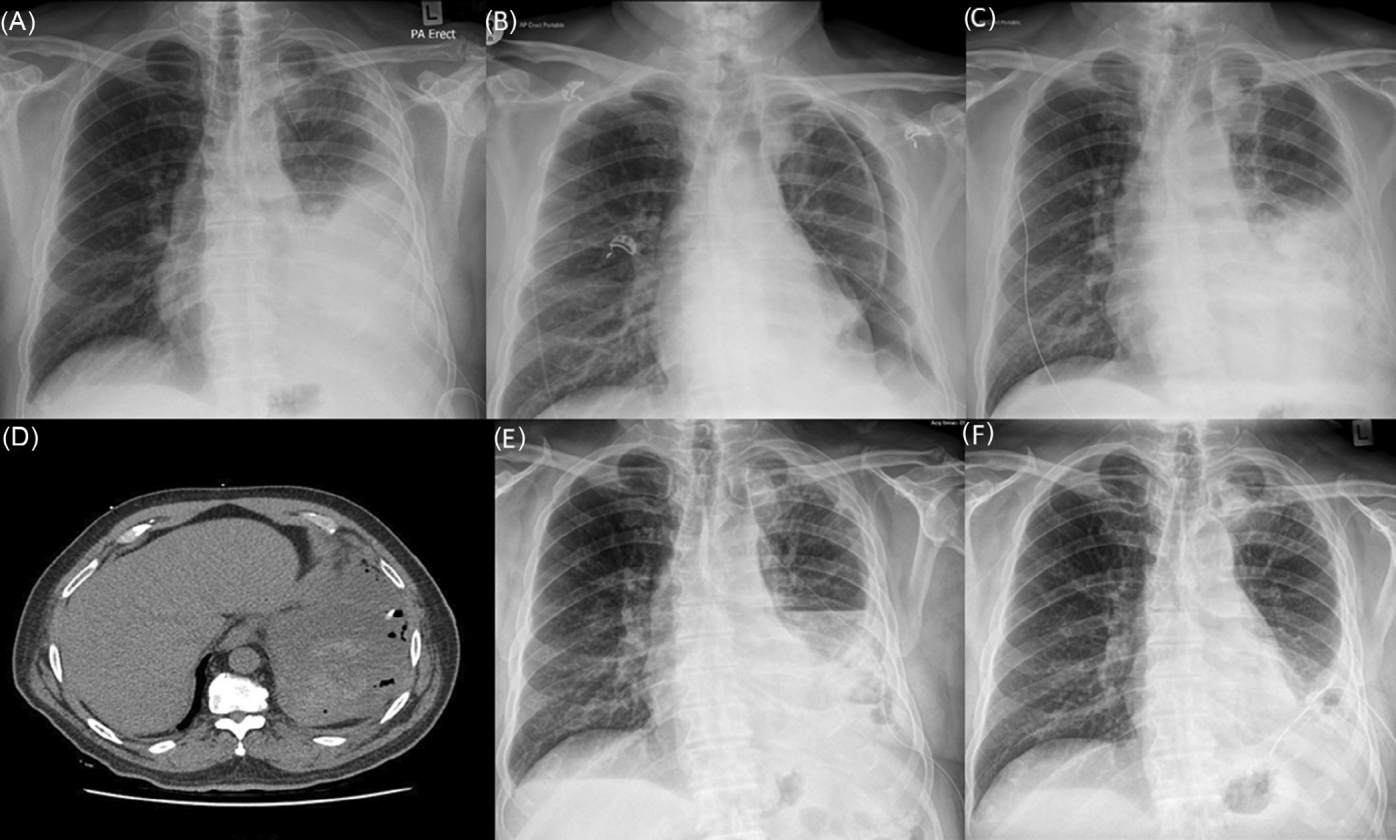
Chest radiograph and computed tomography (CT) images during this patient's treatment course. (A) Pre‐intrapleural fibrinolytics: left pleural effusion; (B) after initial 5 mg intrapleural alteplase with resolution of pleural effusion and evidence of trapped lung; (C) re‐accumulation of pleural fluid 24 hours after 5 mg intrapleural alteplase; (D) CT images showing hyperdense material in the posterior and basal part of the pleural effusion suggestive of haemorrhage; (E) after 1 mg of intrapleural alteplase; (F) chest radiograph after a single 0.25 mg and two 0.5 mg alteplase doses were given with improvement in the left pleural effusion.
